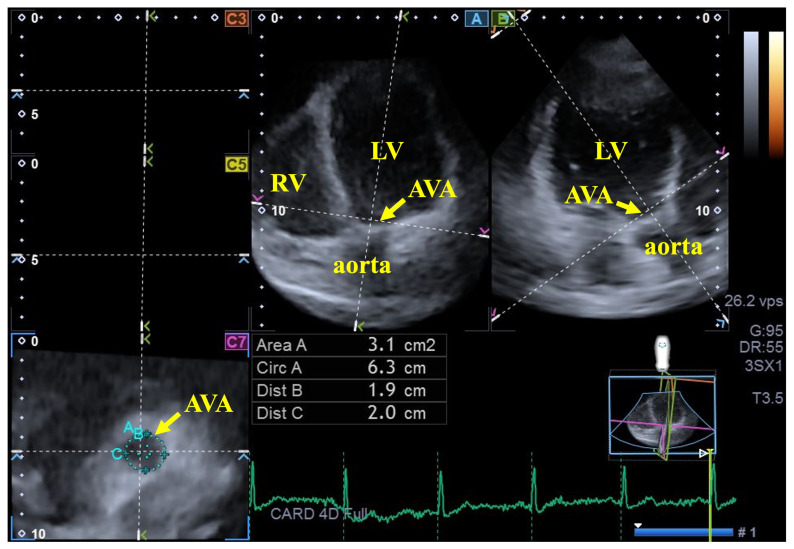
Three-dimensional speckle-tracking echocardiography-derived measurement of the aortic valve annular (AVA) dimensions: apical four-chamber (A) and two-chamber (B) long-axis views optimized on the AVA and ‘en-face’ view of the AVA in C7 cross-sectional view. Abbreviations: LV = left ventricle, AVA = aortic valve annulus, Area = AVA area, Circ = AVA perimeter, Dist B = maximum AVA diameter, Dist C = minimum AVA diameter.
